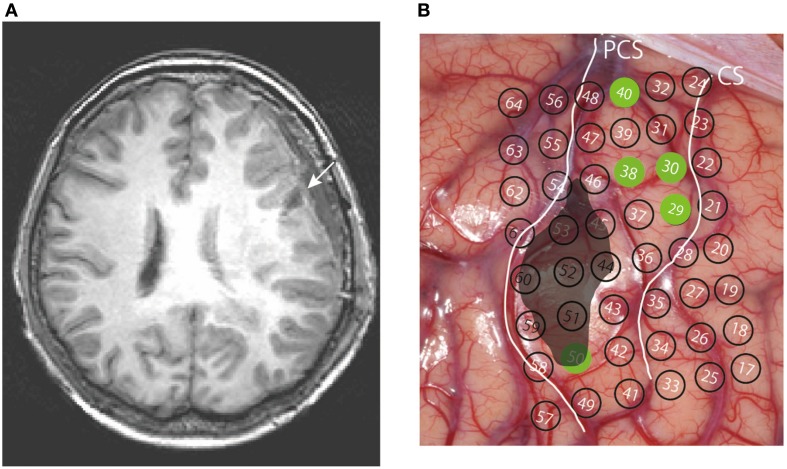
(A) Post-operative axial MR image of the resected region (arrow) in a slice most similar to Figure 1B. (B) Resected area (black) superimposed on the cortical surface, with the positioning of the electrodes.
